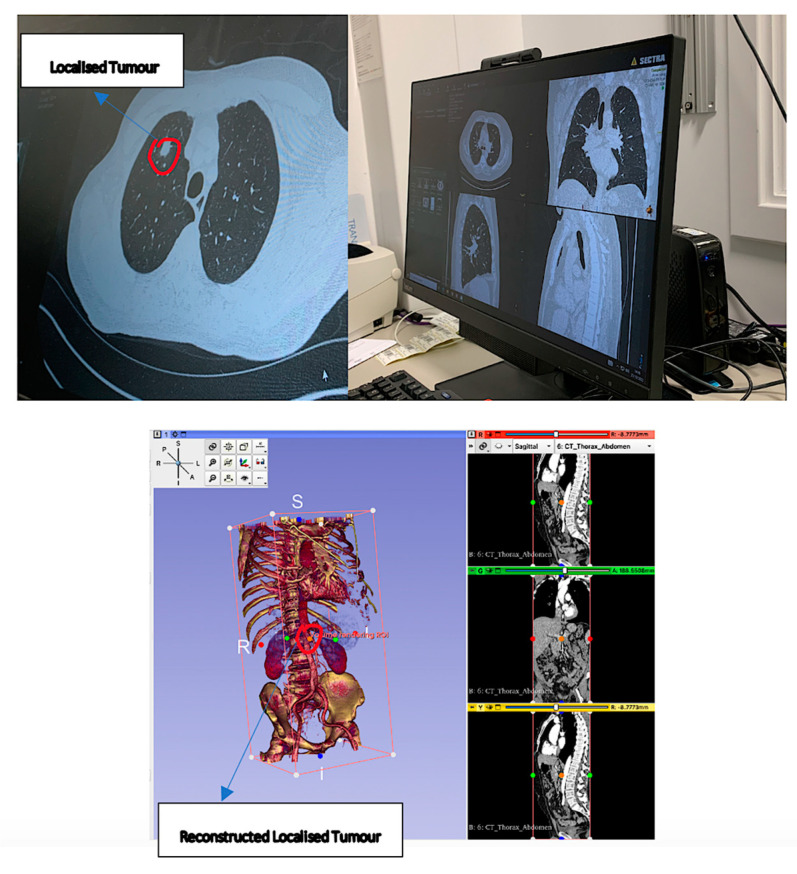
CT scans of the lung with its corresponding 3D reconstruction and marker localization, used by surgeons to locate tumors as indicated by the red marker.
